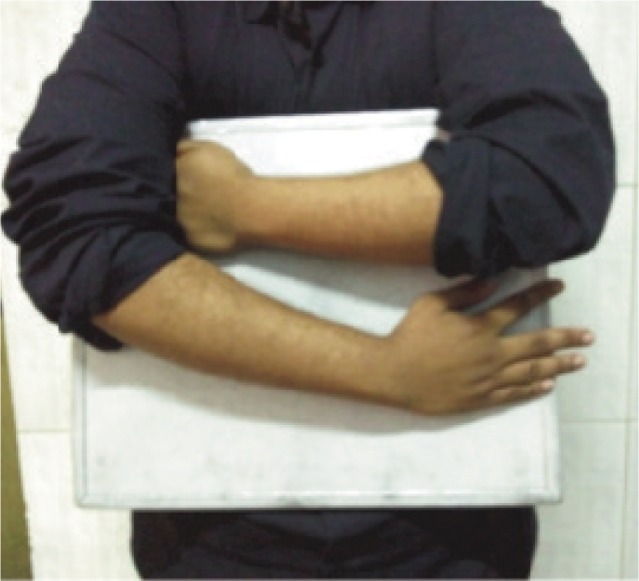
The technique for simultaneous forearm radiography with internal rotation at shoulder.
